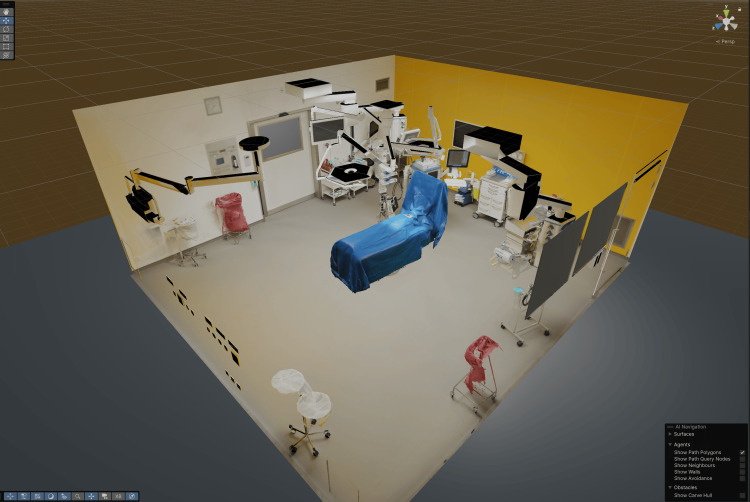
Operating room with patient model in Unity Unity-based virtual reality operating room [18] built from a photogrammetric reconstruction of the Charité - Universitätsmedizin Berlin operating room [19], integrating CT‑derived anatomy models, plus a photogrammetry‑derived patient, positioned and draped for thyroidectomy (hyperextended neck).
